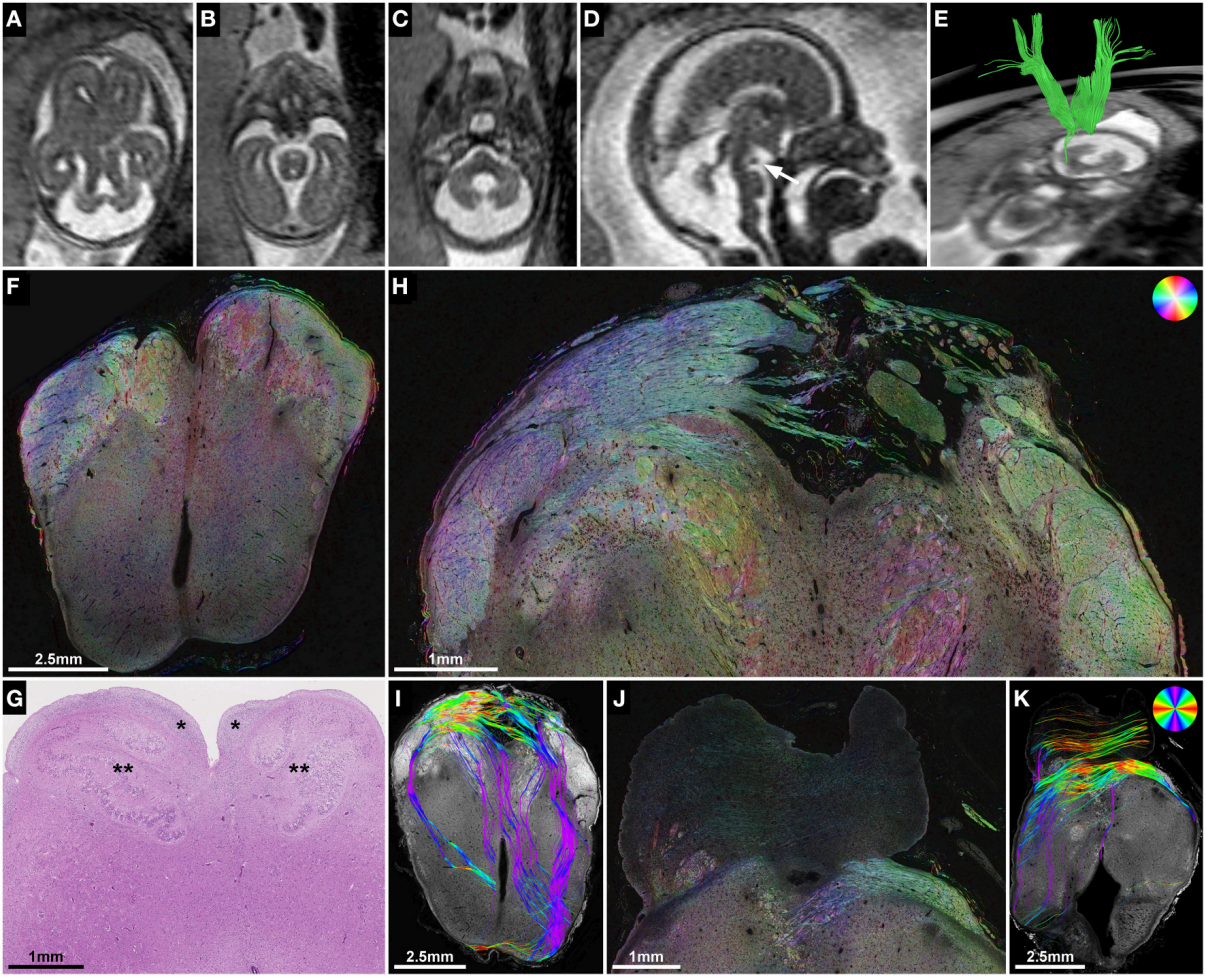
ST analysis of heterotopic corticospinal tract projections in Joubert syndrome. (A–E) Fetal MRI of subject 2 at GW21. Axial T2-weighted images through the brainstem demonstrate molar tooth malformation of the mesencephalon (A) and vermian hypoplasia (C). (D) A midsagittal T2-weighted image shows a nodular hypointense interpeduncular mass (arrow). (E)
